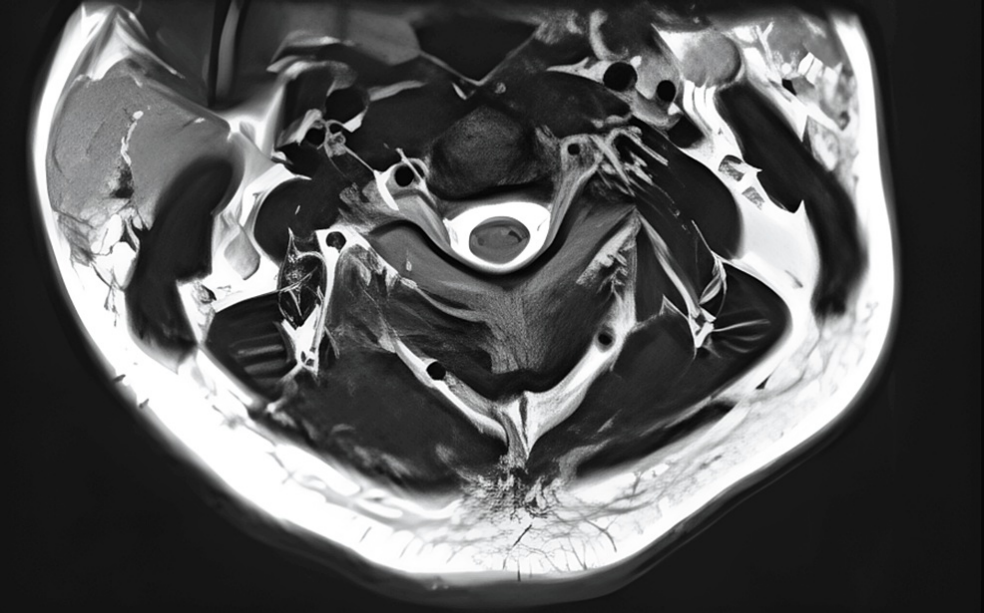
MRI Cervical Spine (T2 Axial view) MRI Cervical Spine: The cervical spinal cord appears normal in diameter and signal intensity. No focal intra or extramedullary lesion is noted.
